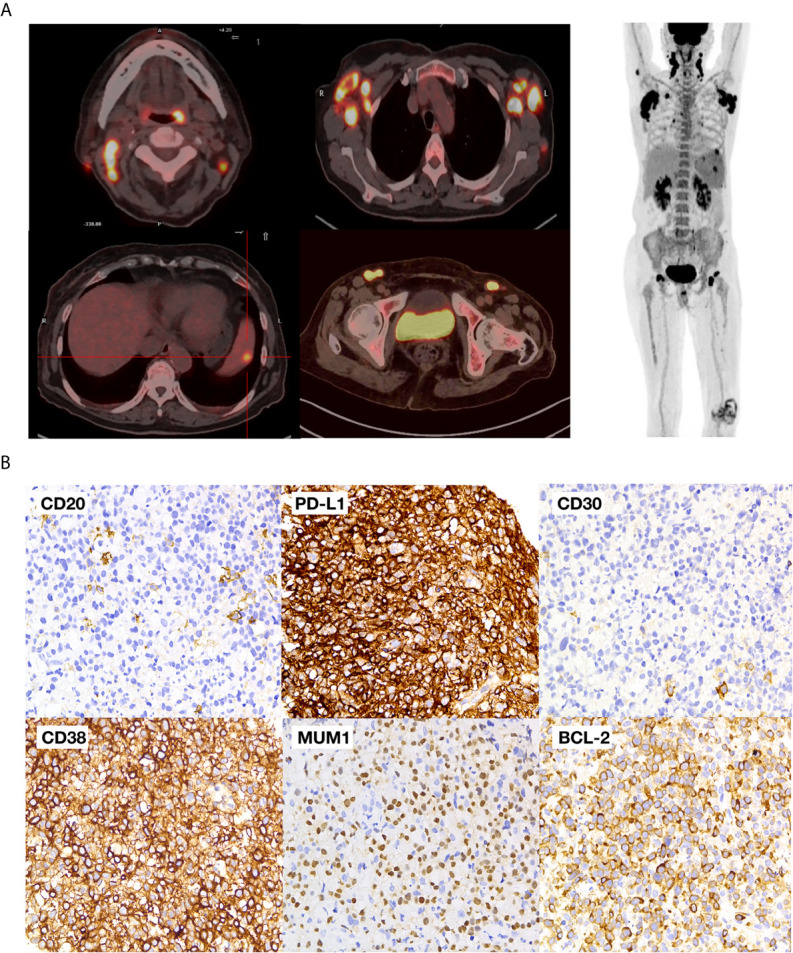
Baseline PET-CT and pathological images (A) PET–CT images of the patient; (B) pathological images of the tumor.
